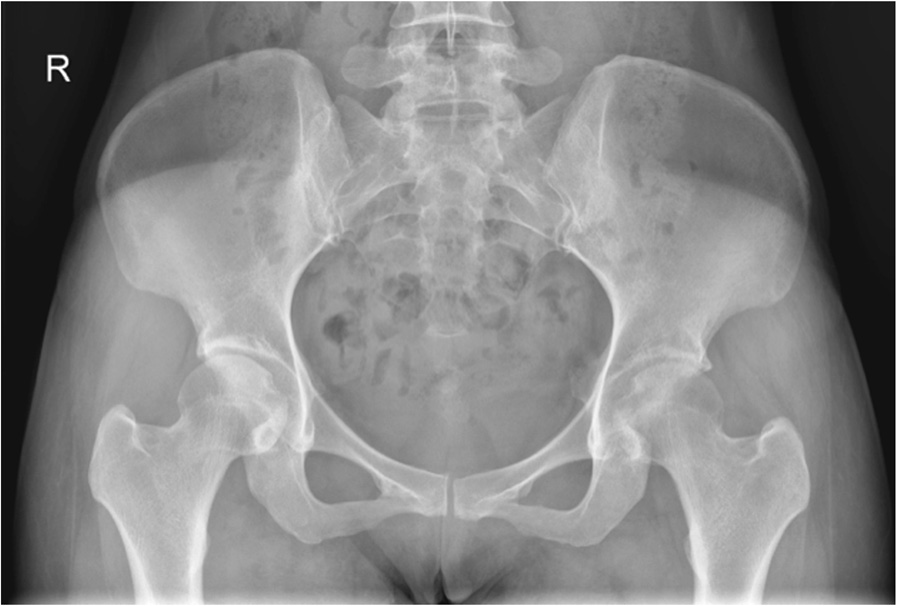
X-ray of her pelvis three months after surgery – Anteroposterior view showed mild osteopenic change over the left femoral head but joint space was preserved.
